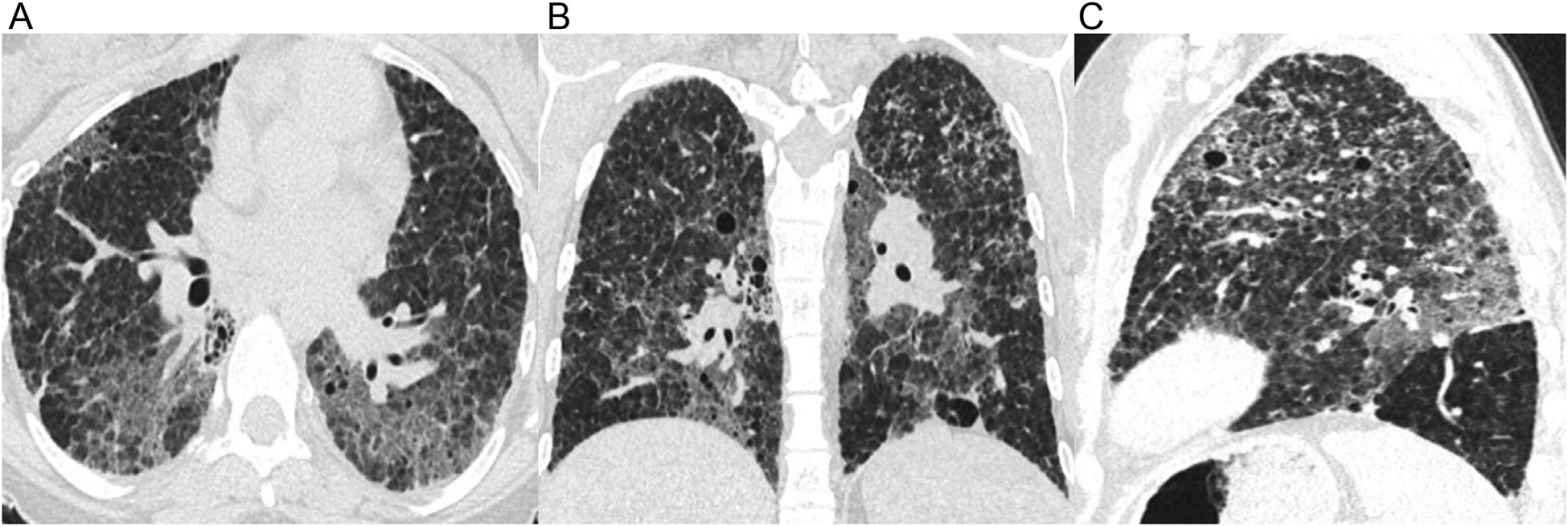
Imaging findings at 32 years of age (case 2). Axial (A), coronal (B), and sagittal (C) chest CT images display worsening findings, including patchy ground glass opacities with interlobular septal thickening, thin-walled cysts, and reticulations.
